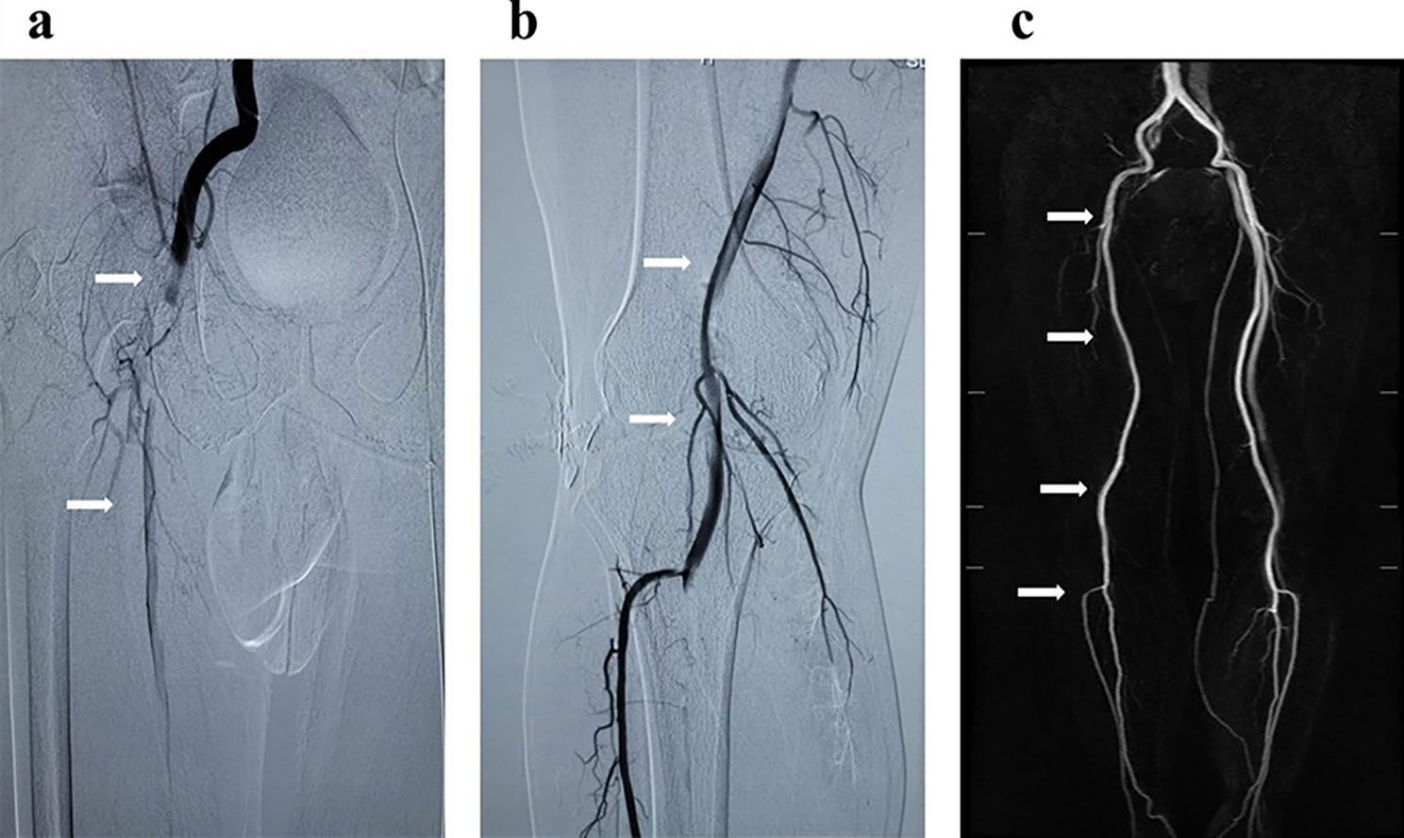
Arteriogram of the right lower extremity showed a complete occlusion with a filling defect of the femoral artery (a, arrows) and popliteal artery (b, arrows), and MRI without contrast agent (c, arrows) showed disappearance of the right femoral-tibial thrombosis after thrombectomy and thrombolysis
